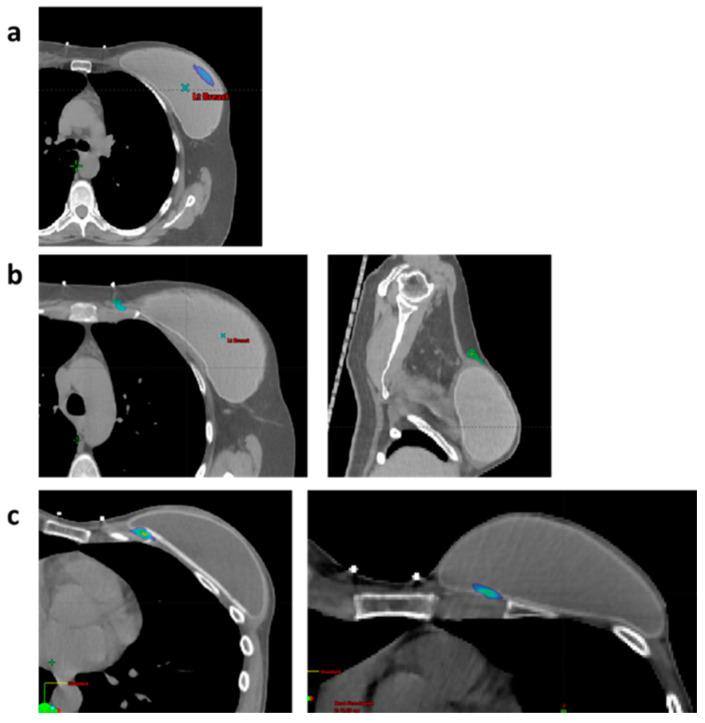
Hotspot type with respect to the reconstructed breast. Representative CT scans demonstrating three types of hotspots: inside the implant (a), outside the implant (b), and overlap respective to the implant and chest wall (c).
